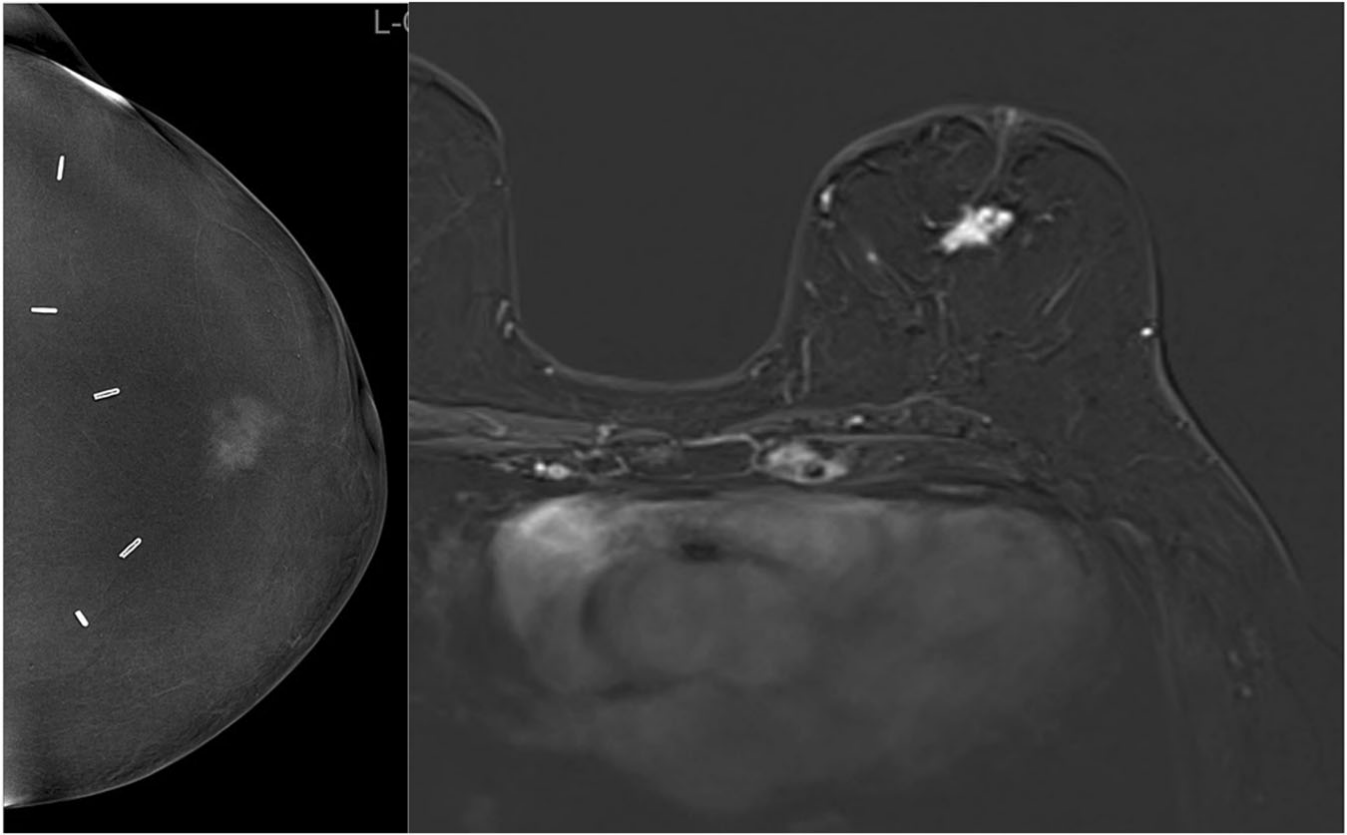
58-year-old woman with a local recurrence 8 years after the first diagnosis (triple-negative breast cancer). The lesion is clearly visible in both modalities, but conspicuity was rated higher on CE-MRI
